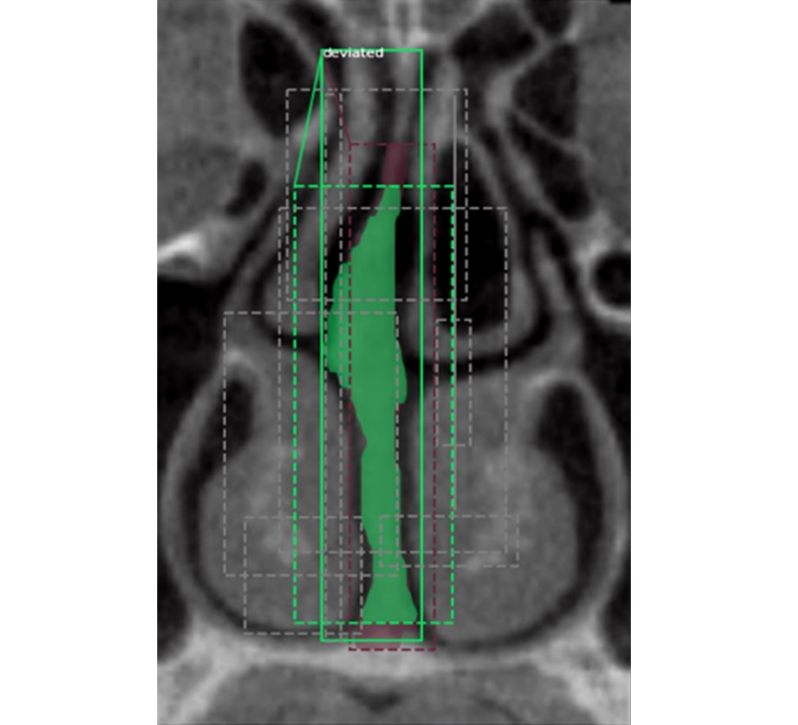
Region proposals in the cropped cone beam computed tomography image showing potential areas of interest (10 random Regions of Interest out of 200).
